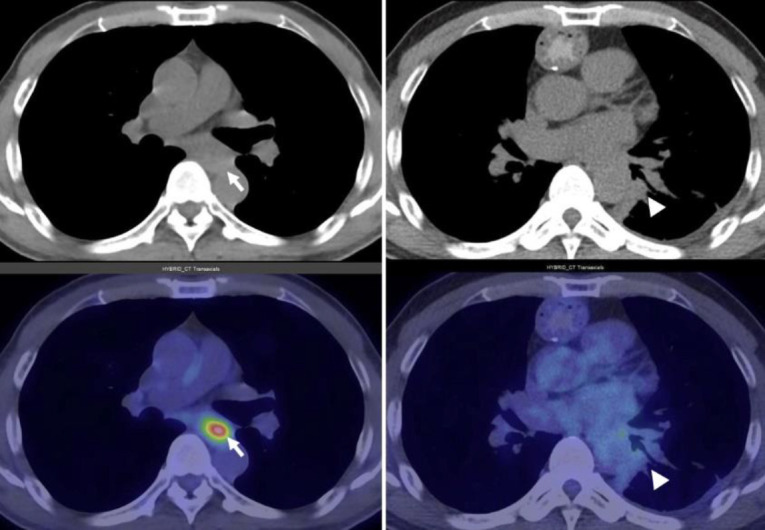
(A) Axial ^18^F-FDG PET/CT images from the initial staging scan (August 2022) demonstrate ^18^F-FDG-avid uptake in the mid-esophagus (arrow), corresponding to the primary tumor. (B) Follow-up ^18^F-FDG PET/CT performed in March 2025, after the patient had undergone esophagectomy with gastric pull-up reconstruction, shows a mildly hypermetabolic lesion in the left lower bronchus (arrow head), located adjacent to the previous tumor bed, suggestive of intraoperative tumor cell seeding
